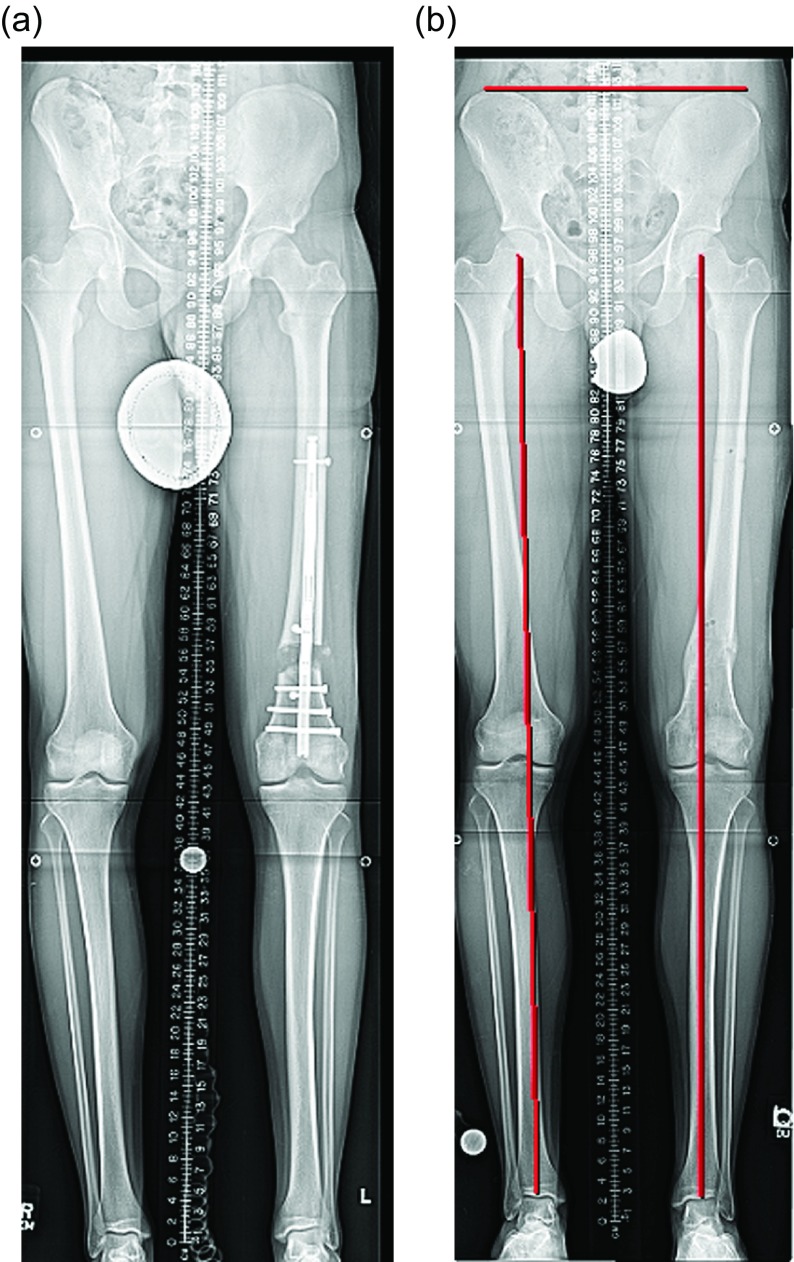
(a) The lengthening is complete and the limb length is checked. The regenerate bone is filling in but is still plastic enough to allow for fine-tuning of the length. The long-standing X-ray can be affected by hip and knee joint muscle contractures yielding a false length measurement. This needs to be considered before over-lengthening the limb. (b) The final alignment and length are checked after ILN removal.
